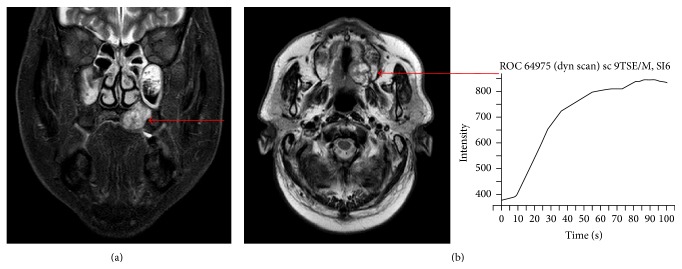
MR images. (a) Frontal plane section. (b) Horizontal section. CE-MRI showed that the mass extended upward and invaded the nasal cavity. The mass showed hypointensity on T1-weighted images, hyperintensity on T2-weighted images, and an irregular margin with internal enhancement. Dynamic MRI revealed a plateau type lesion that was suspected to be a malignant tumor.
